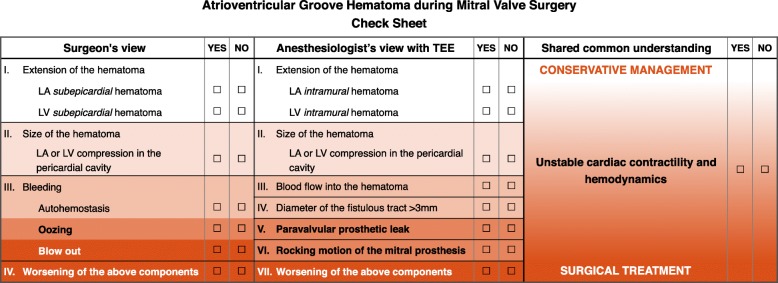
Original check sheet for intraoperative atrioventricular groove hematoma. The check sheet was designed for reasonable intraoperative decision-making based on two complementary viewpoints: the surgeon’s view of the surgical field and the anesthesiologist’s view using transesophageal echocardiography. Multidisciplinary severity stratification and a shared common understanding among surgical team members are essential when facing unstable and uncertain situations
